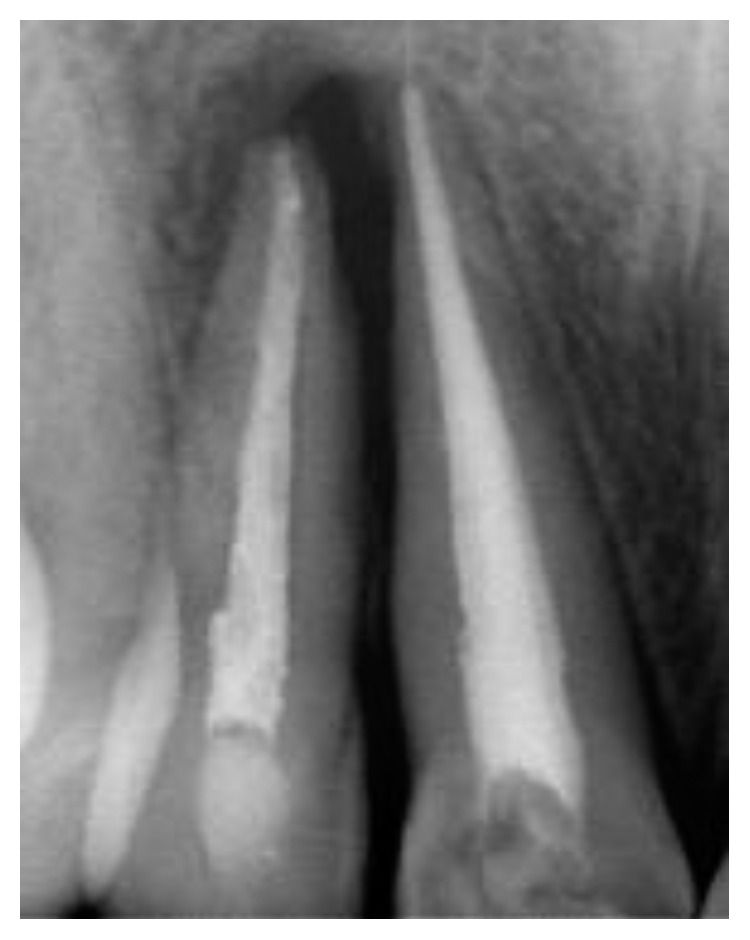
Radiograph of control after 3 months.
